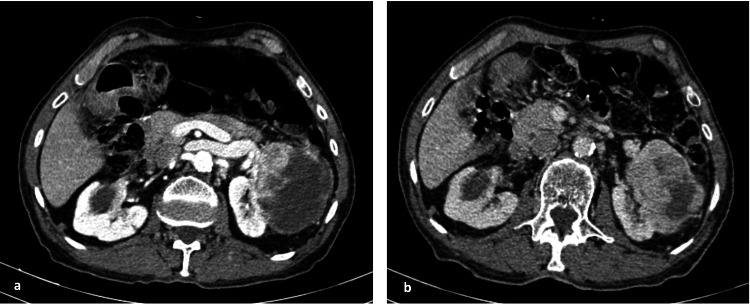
CT scan imaging showing significant tumor regression a: before treatment; b: after six months of treatment
